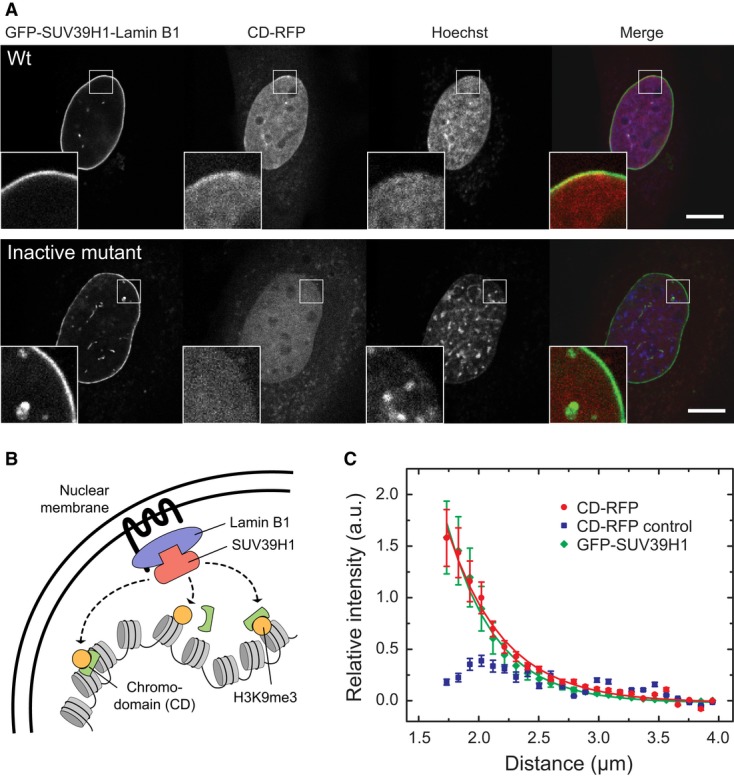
Propagation of H3K9me3 by stably tethered SUV39H1 to nucleosomes in its spatial
